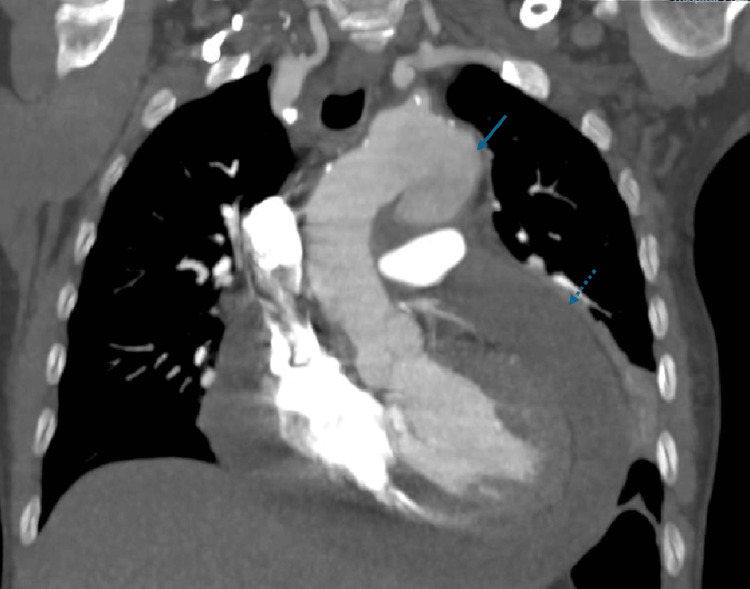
Thoracic CT angiography demonstrating a saccular aortic aneurysm (solid arrow) adjacent to the aortic arch (44×25×39mm) and a homogeneous pericardial effusion (dashed arrow) up to 3 cm in thickness. CT: computed tomography
